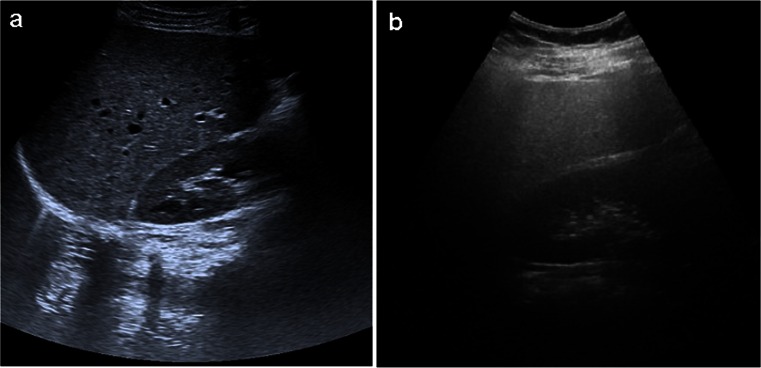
Abdominal US of a patient without NAFLD (a) in comparison with a patient with NAFLD (b). US scan of the liver of a 26-year-old patient without NAFLD showed solid homogenous echo texture (a). US scan of the liver of a 45-year-old patient with NAFLD showed diffusely increased liver echogenicity, blurring of vascular, and increased acoustic attenuation (b). *US* ultrasonography
